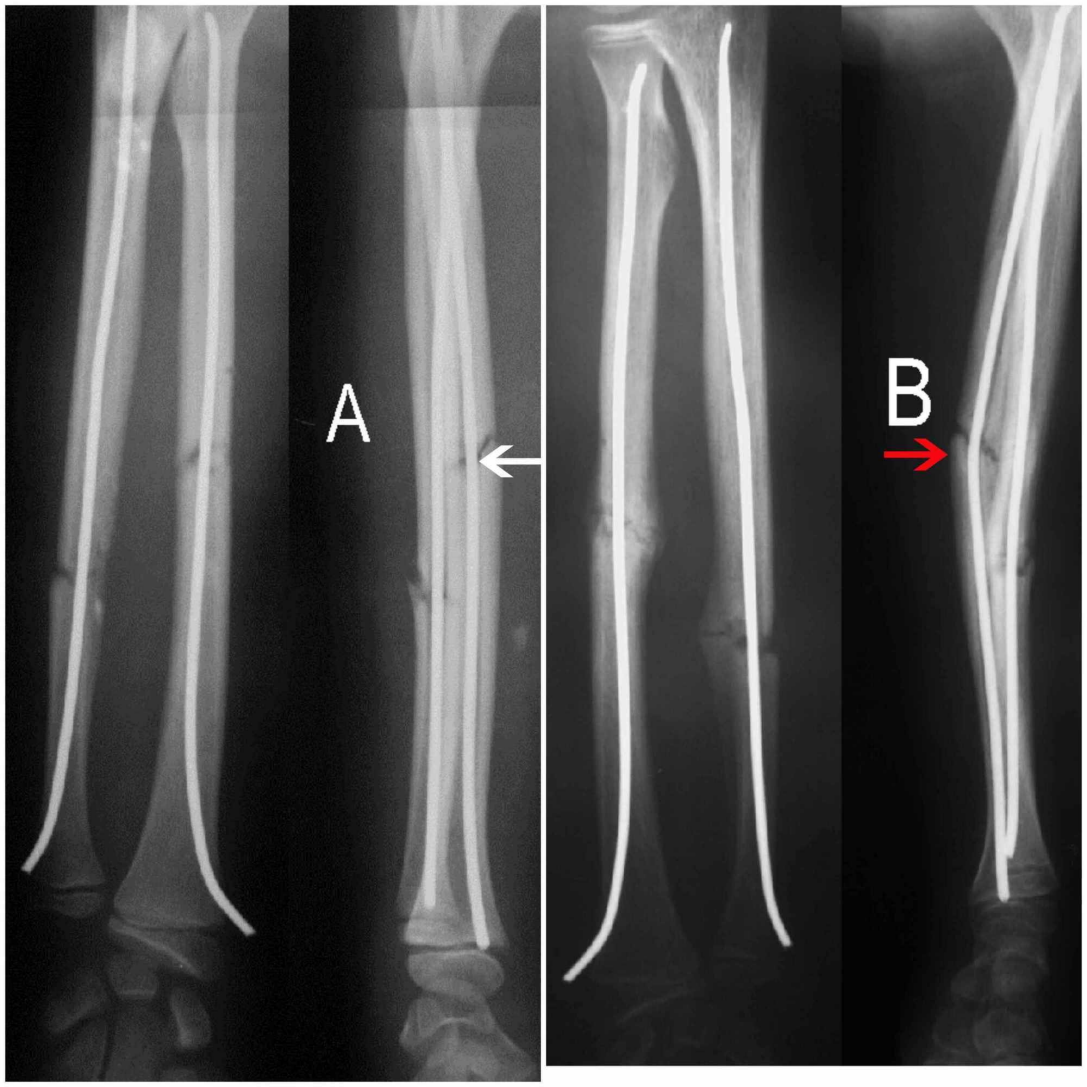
Angulation due to bending of the nail (A) Immediate postoperative X-ray showing acceptable reduction of the radial fracture and no angulation (white arrow) evident in the lateral (L) view. (B) One-month postoperative X-ray showing angulation of the radial fracture due to bending of the nail (red arrow) evident in the L view, in a case where no cast was used, and the patient suffered a new injury.
